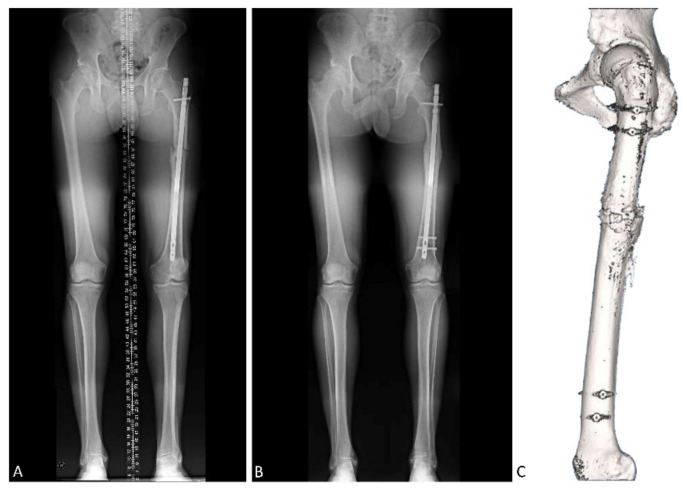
Pre- and postoperative image study (Patient 3). Teleradiographs are conducted to observe rotation before (A) and after surgery (B). It also indicates callus formation over time. The CT scan also indicates whether the alignment of the femur is correct after surgery (C).
